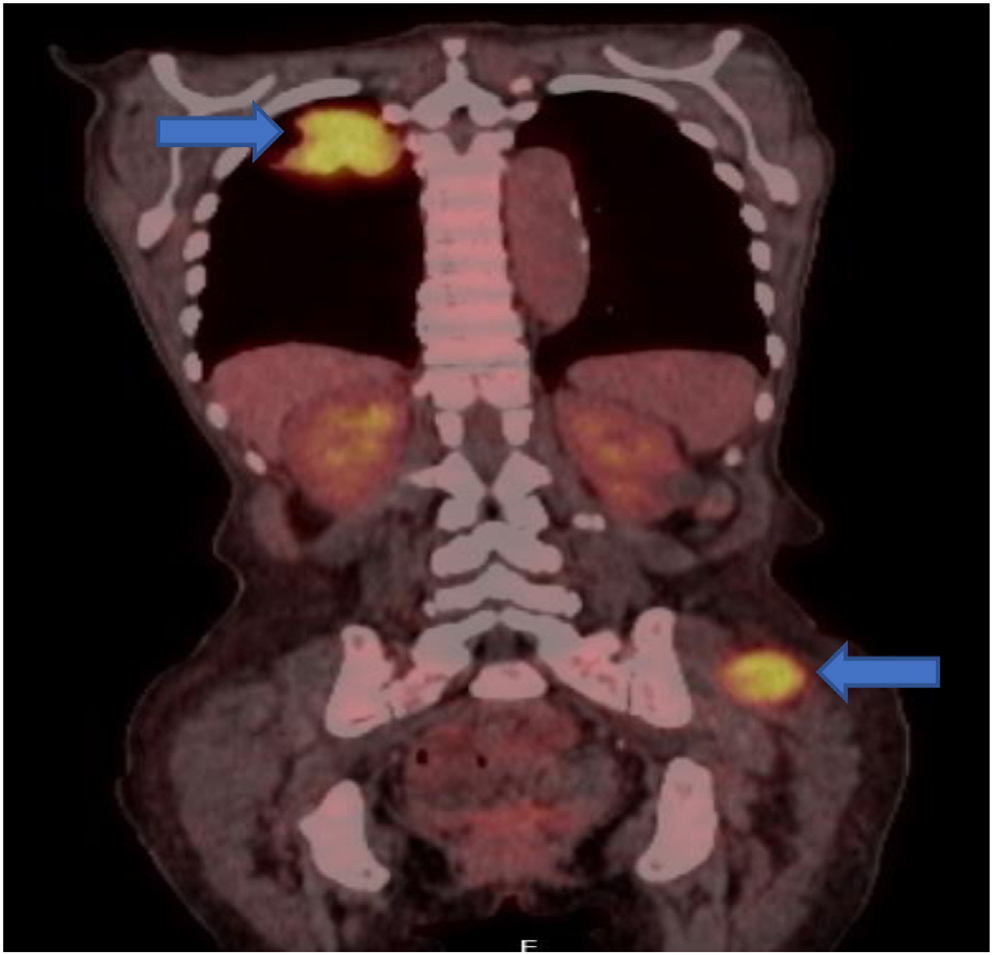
^18^FDG-PET coronal CT displaying two avid lesions, in the right upper lobe and left gluteal region.
